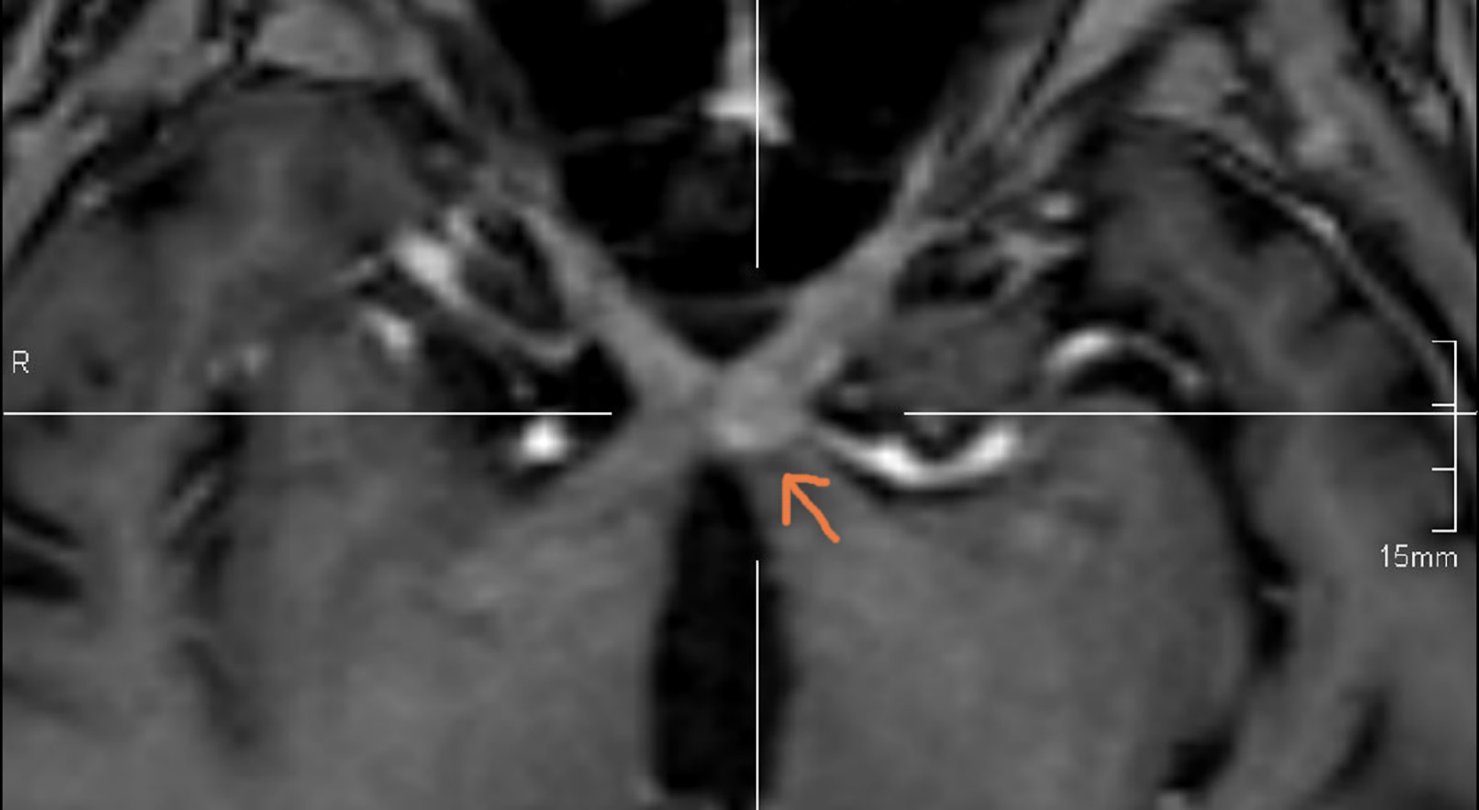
Preoperative MRI, axial T1 with contrast, showing the lesion of the chiasma.
